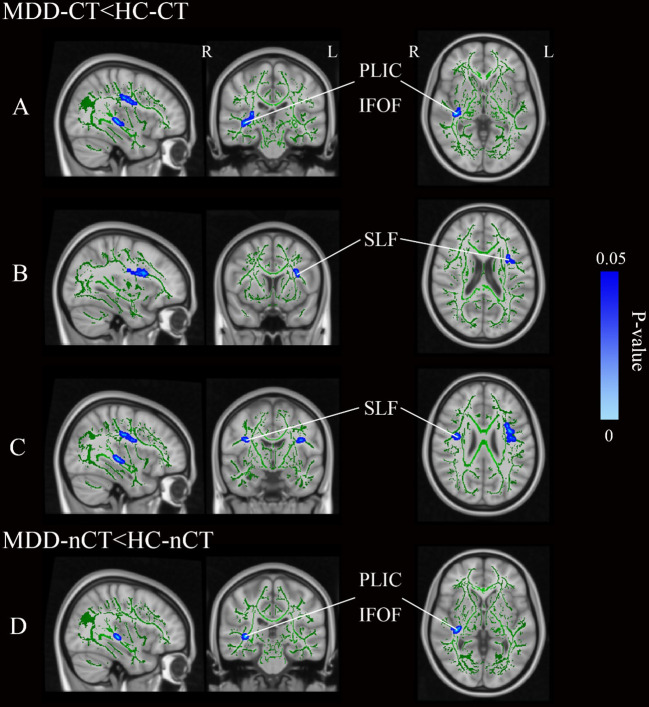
(A–C) Represent three distinct cluster regions where significant differences between MDD-CT and HC-CT were observed following simple effects analysis. (D) Represents a distinct cluster region where significant differences between MDD-nCT and HC-nCT were observed following simple effects analysis. Blue regions represent tracts with decreased FA (MDD-CT vs HC-CT and MDD-nCT vs HC-nCT). MDD, major depressive disorders; HC, Healthy Controls; CT, childhood trauma; FA, fractional anisotropy; L, left hemisphere; R, right hemisphere; SLF, superior longitudinal fasciculus; PLIC, posterior limb of the internal capsule; IFOF, inferior fronto-occipital fasciculus.
